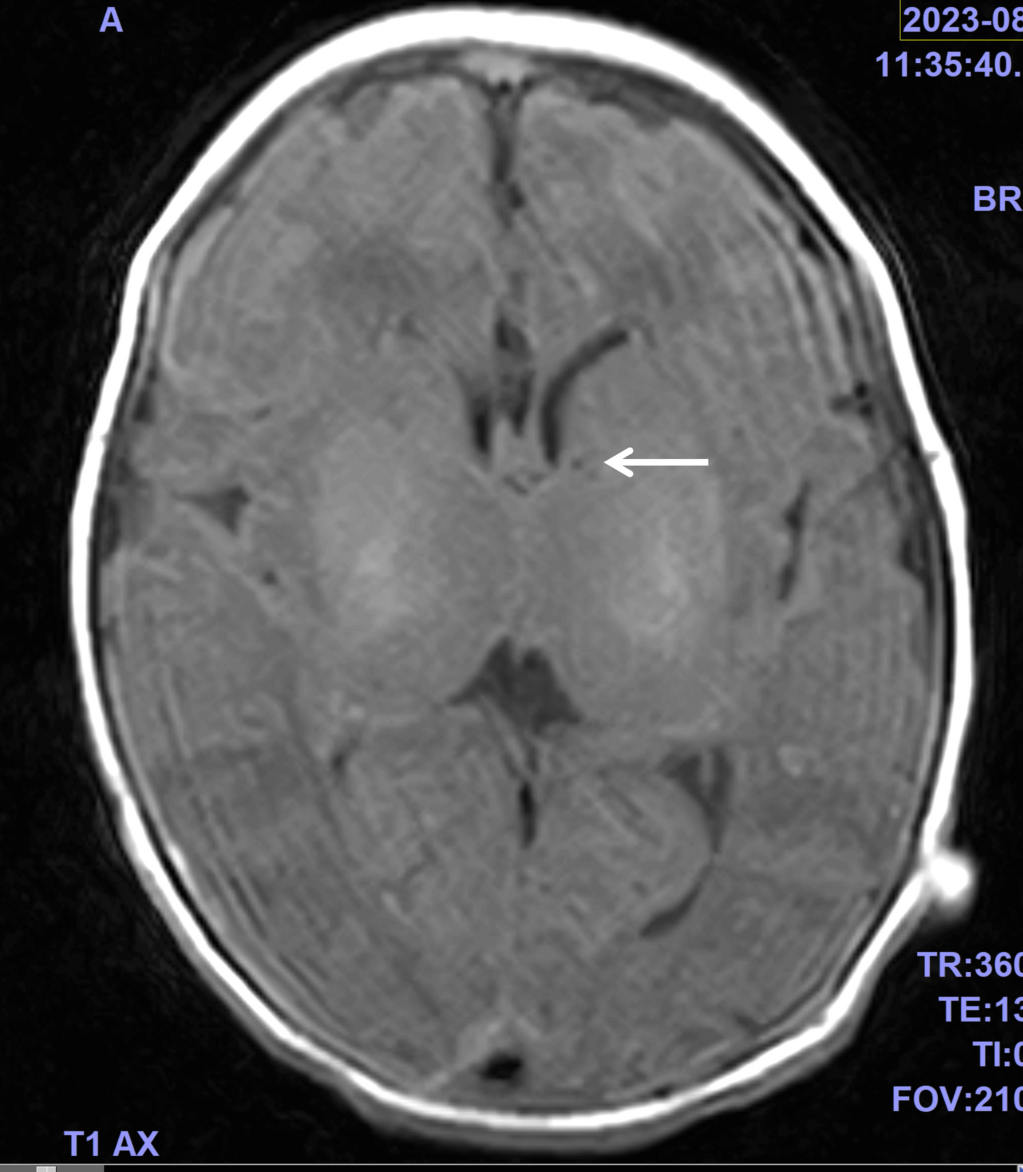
Axial T1-weighted magnetic resonance imaging of the brain Axial T1-weighted magnetic resonance imaging of the brain showed no abnormal findings except for subependymal cysts (indicated by a white arrow).
